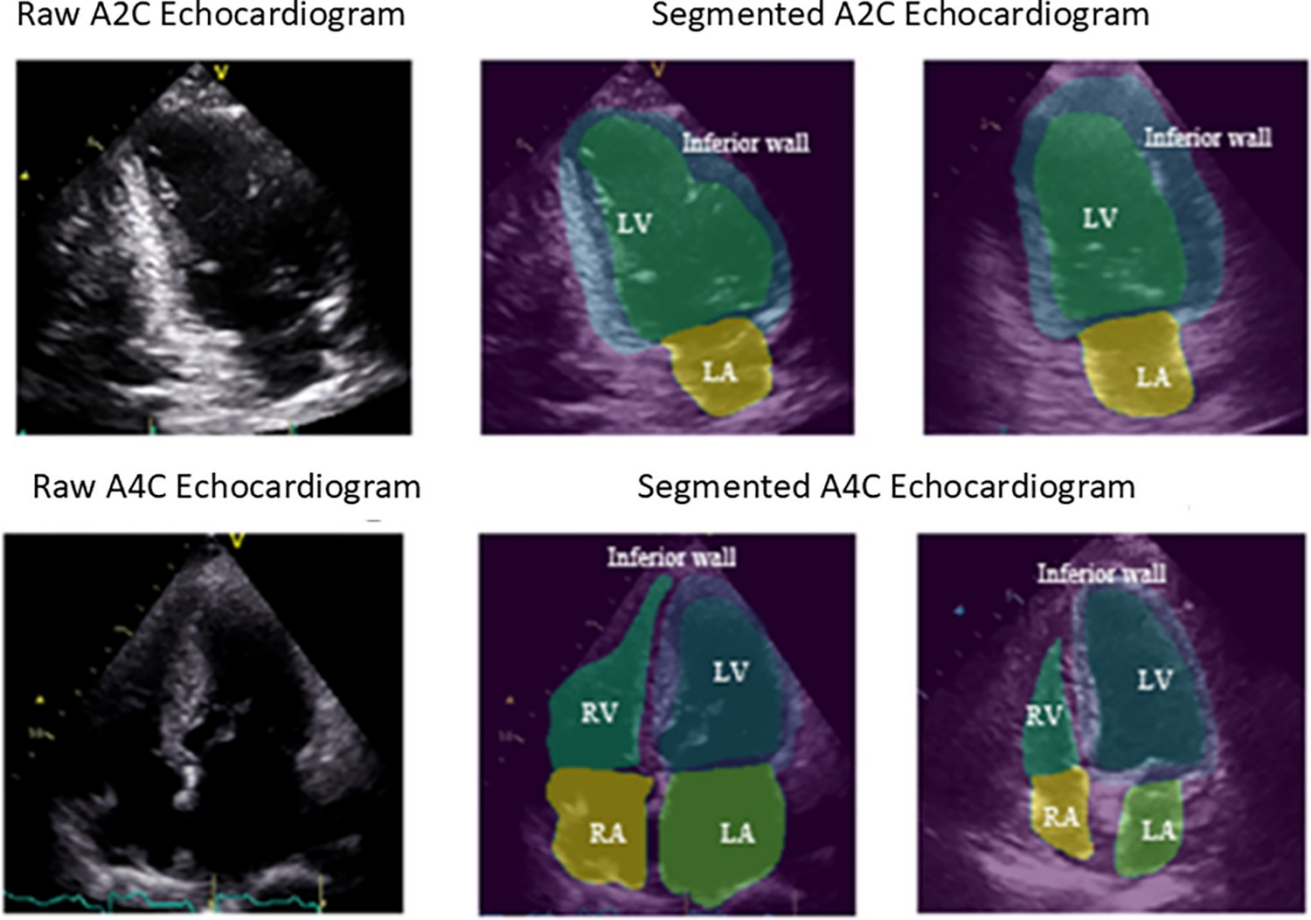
ROI of cardiac structure in echocardiograms generated by U-Net.
